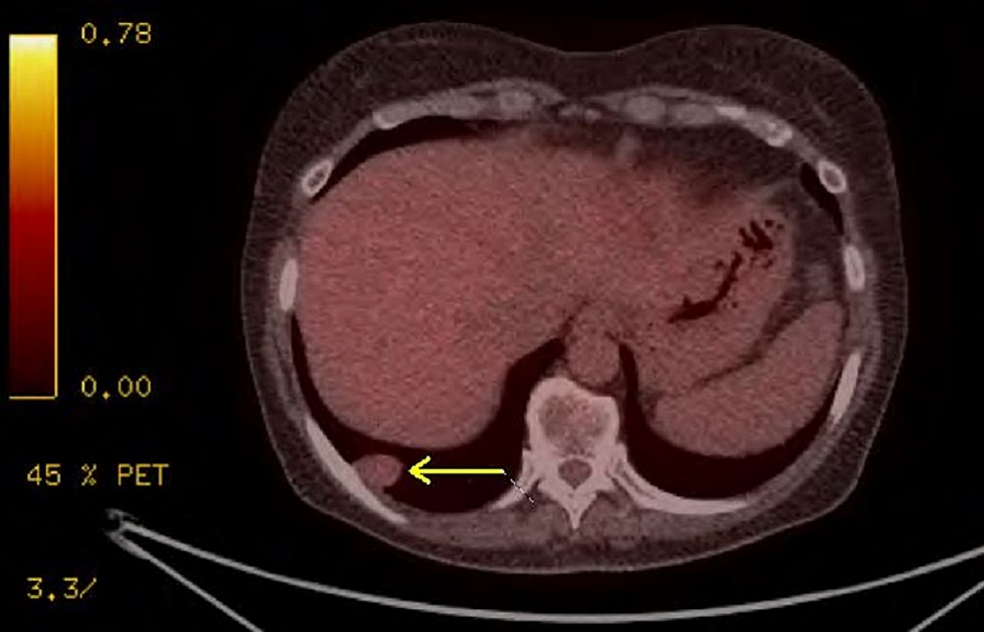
CT/PET of the chest in the axial view demonstrating a 2.5 x 1.7-cm lobulated non-calcified mass (arrow) in the right posterior sulcus with faint FDG-avidity of SUVmax of 2.127 CT: computed tomography; PET: positron emission tomography; FDG: fluorodeoxyglucose; SUVmax: maximum standardized uptake value
